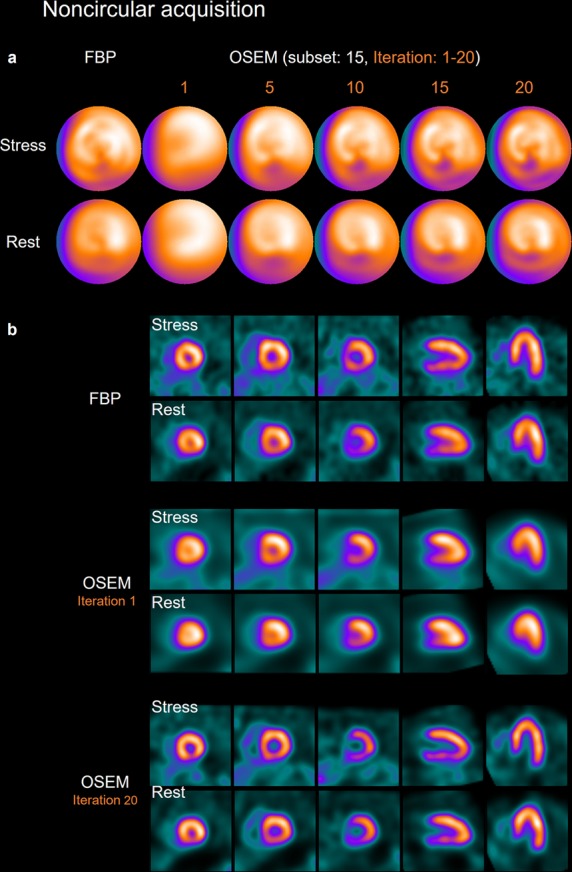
Clinical stress-rest polar maps (a) and SPECT images (b) in a 60-year-old male patient with slight ischemia in the inferior wall. Height and weight were 167 cm and 66 kg, respectively. All images were acquired with the noncircular orbit of gamma cameras. The Iterations changed from 1 to 20, and a subset was constantly 15.
